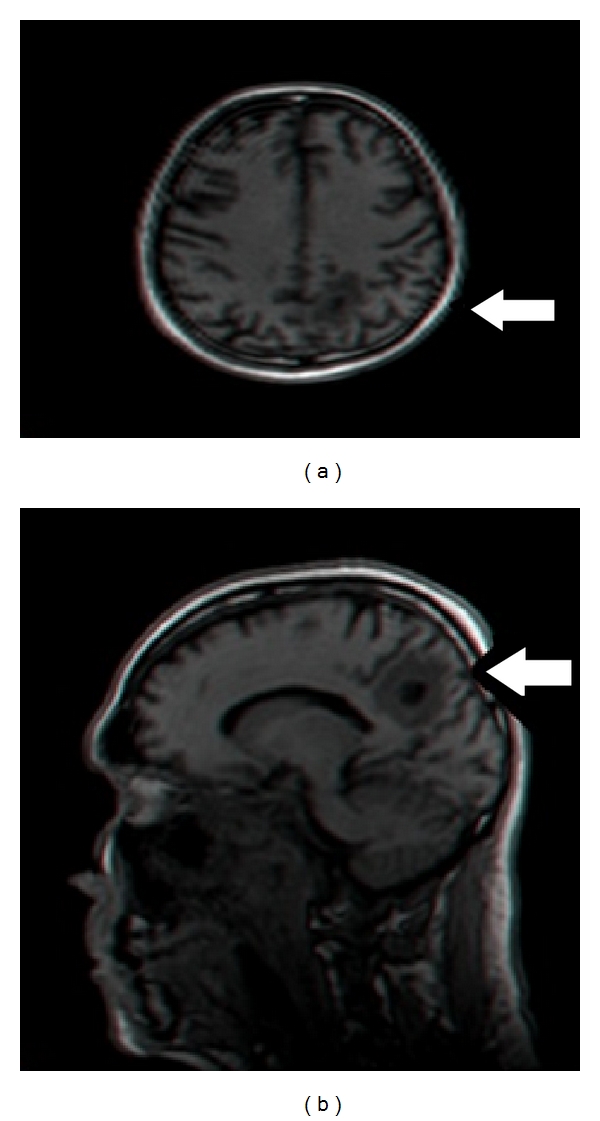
A Brain MRI showed a single occipital brain lesion.
